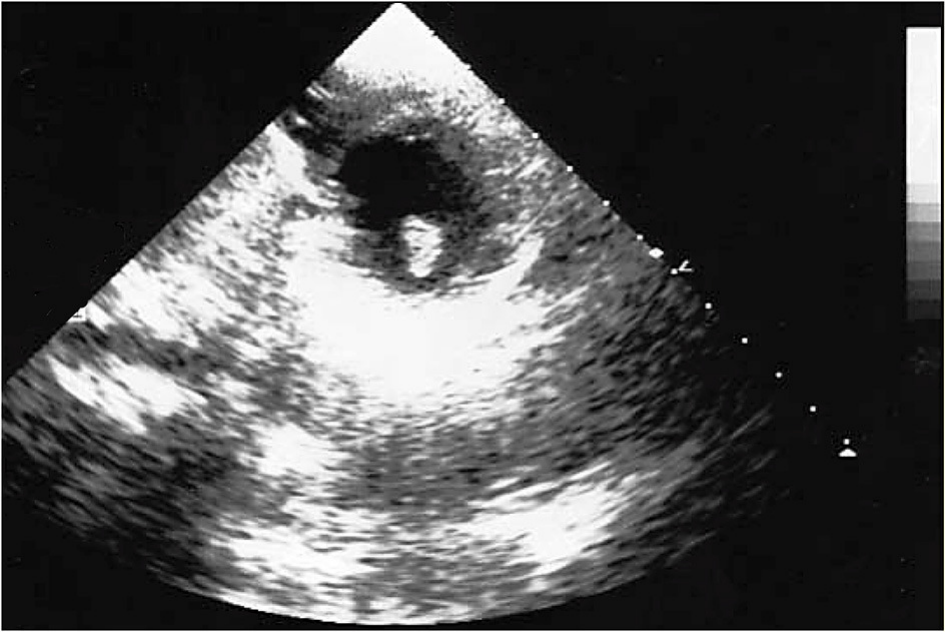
Echocardiographic image: solid neoplasm floating in the left ventricle outflow chamber, connected to the anterolateral papillary muscle of the left ventricle and characterized by homogeneous density, large base implant, and regular margins.
